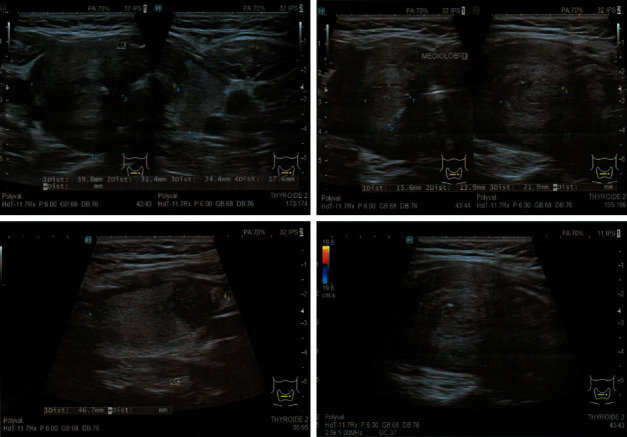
Patient's cervical ultrasound showing a hypovascularized nodular goiter.
